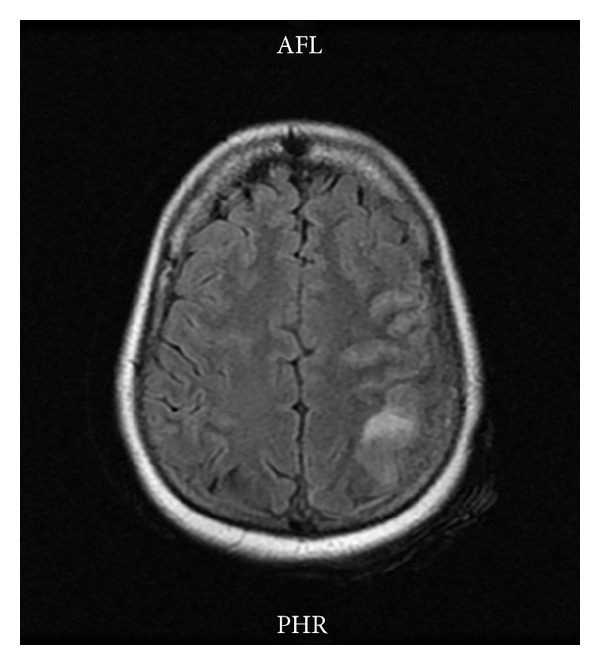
MRI brain T2W axial image showing hyperintense left parietal lesion.
